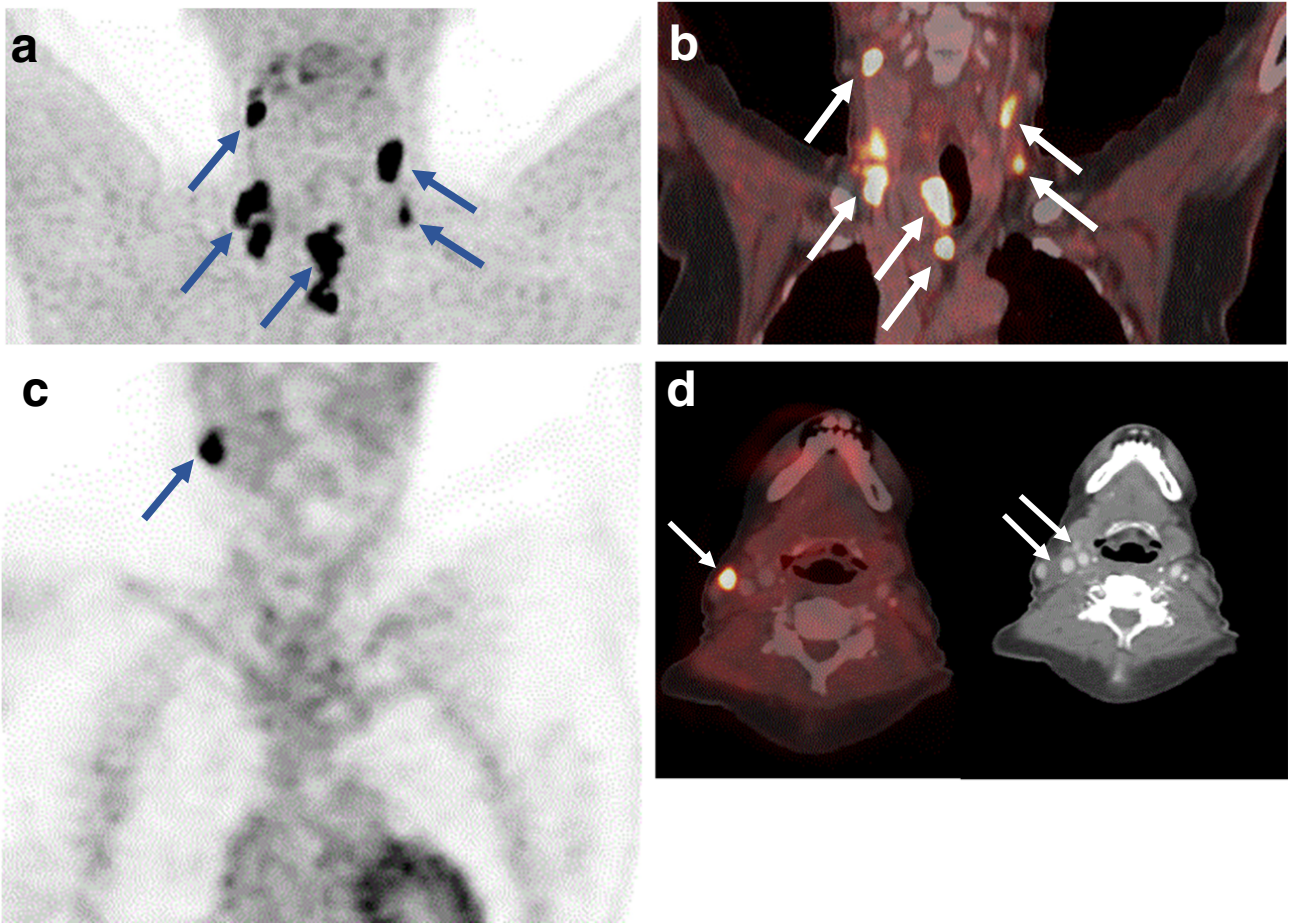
A 65-year-old female patient with MTC and bilateral lymph node metastases with persistently elevated levels of serum basal calcitonin after surgery. a, b Preoperative [^18^F]DOPA PET/CT images show DOPA-positive primary tumour in the right thyroid lobe with bilateral DOPA-positive lymph nodes in the cervical region (levels II, III and IV) and central region (level VI). c, d [^18^F]DOPA PET/CT images in the same patient 2 months after total thyroidectomy, and central and bilateral lymph node dissection, show a DOPA-positive residual lymph node in the upper right cervical region (level II)
